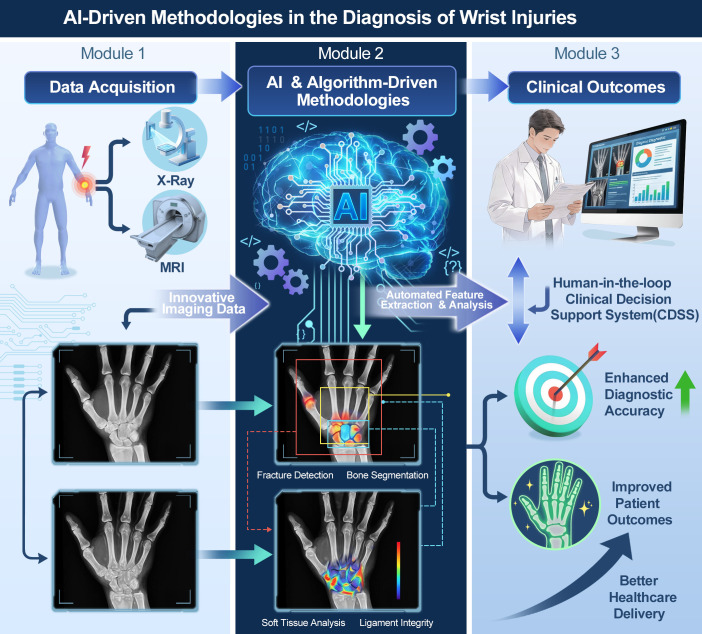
AI Technologies in Wrist Injury Diagnosis. The process begins with data acquisition from X-ray and MRI, followed by AI-based feature extraction and analysis, ultimately leading to improved diagnostic accuracy, patient outcomes, and healthcare delivery.
